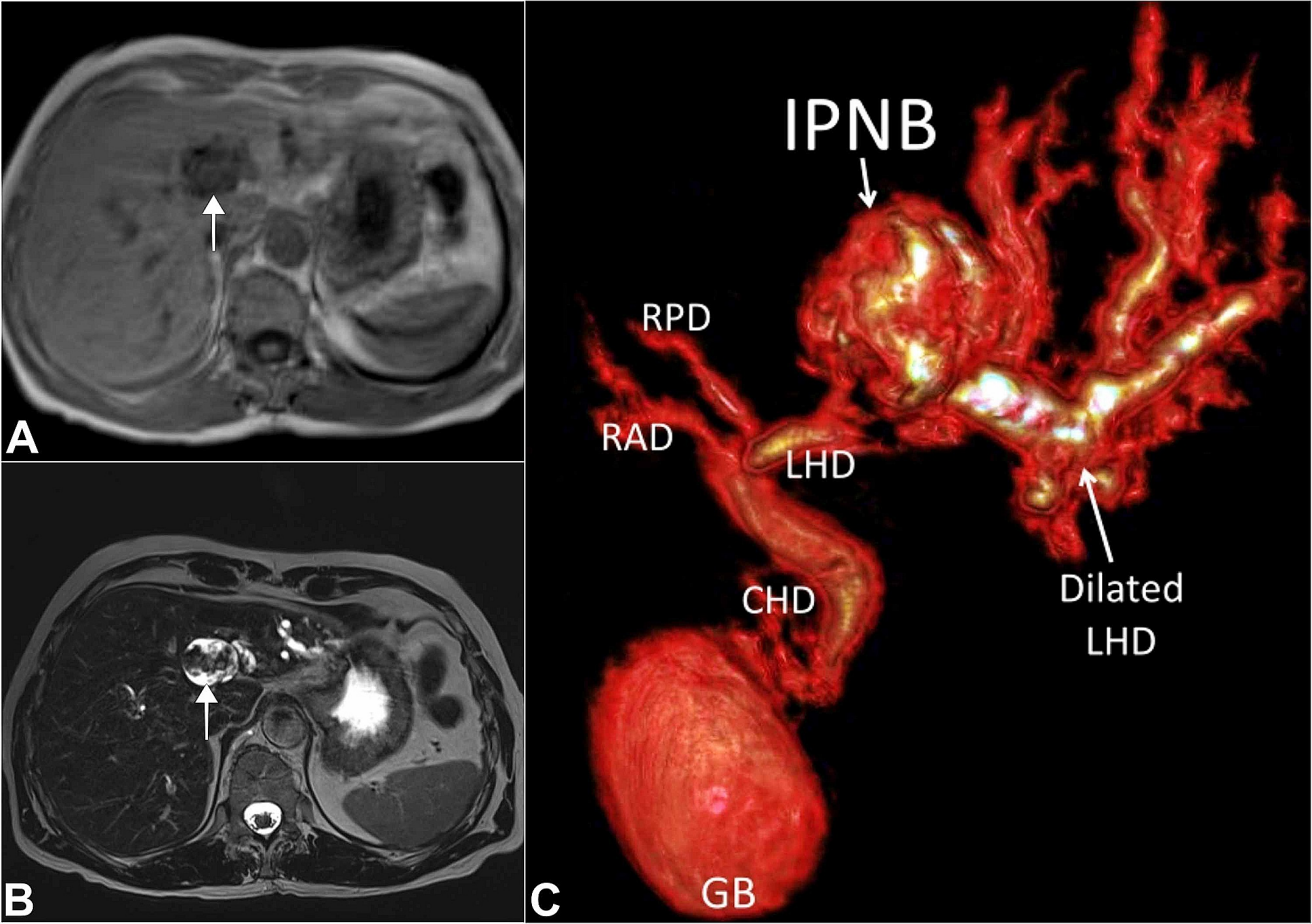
MRI images. (A) T1-weighted axial section with low to isointense lesion (white arrow); (B) T2-weighted axial section with hyperintense lesion with intraductal papillary projections (white arrow); (C) 3D volume reconstructed MRCP image showing the cystic lesion with communication with LHD which is causing proximal dilatations of the biliary ducts. MRI, magnetic resonance imaging; MRCP, magnetic resonance cholangiopancreatography; RAD, right anterior sectoral duct; RPD, right posterior sectoral duct; GB, gall bladder; LHD, left hepatic duct
